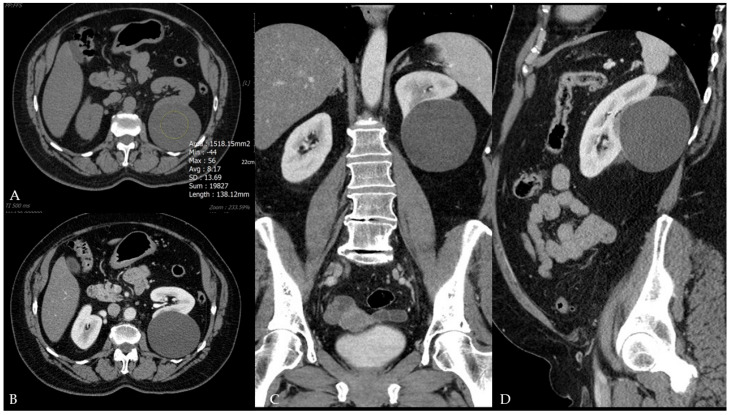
Computed tomography of the abdomen and pelvis (year 2015): (A)—Non-contrast-enhanced image in the axial plane; a large, round, and partially extracortical cystic lesion is seen in the lower pole of the left kidney, measuring up to 8 cm in diameter (measurement lines are not depicted) with no internal septa or solid component. The lesion contains water content [i.e., at the internally placed region of interest (ROI)] measuring around 8 Hounsfield units. (B)—A contrast-enhanced image in the axial plane; the same lesion demonstrates no enhancement following contrast media administration. (C,D)—Contrast-enhanced images of the same lesion in the coronal and sagittal planes confirm its completely benign morphology, correlating with a Bosniak type I cystic lesion.
